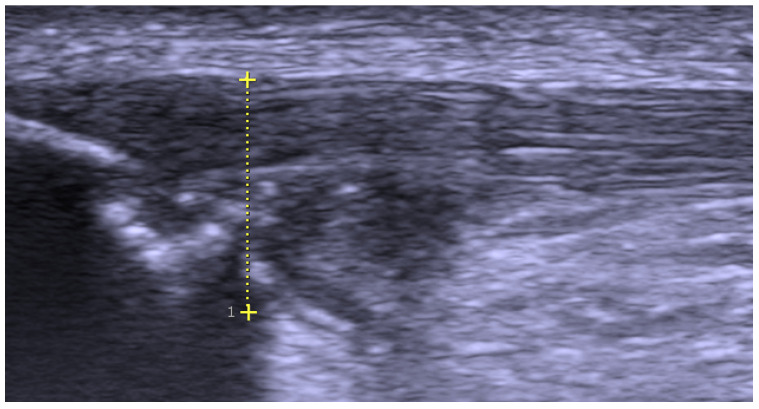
Greyscale ultrasound examination—longitudinal view of a patient with proximal patellar tendinopathy. There is a thickening of the proximal patellar tendon (marked) including tendinopathy on the dorsal side and a suspected partial rupture in close relation to a sharp bone edge in the patellar tip.
